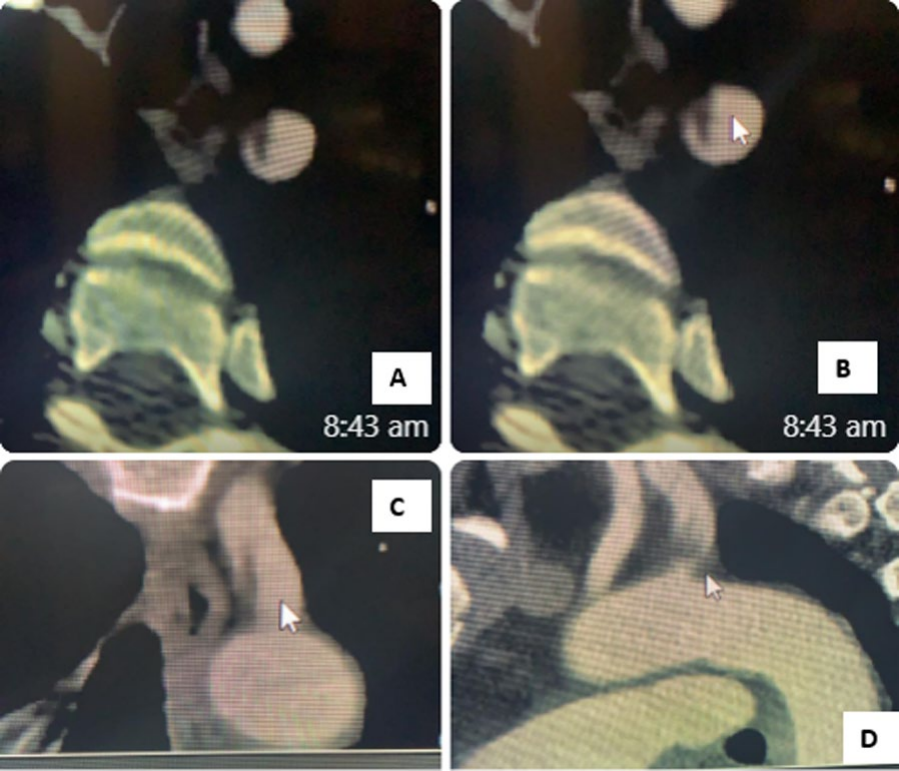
Multi-slice CT angiography A and B axial, C coronal and D sagittal images that demonstrate a dissection flab at the origin of left subclavian artery
